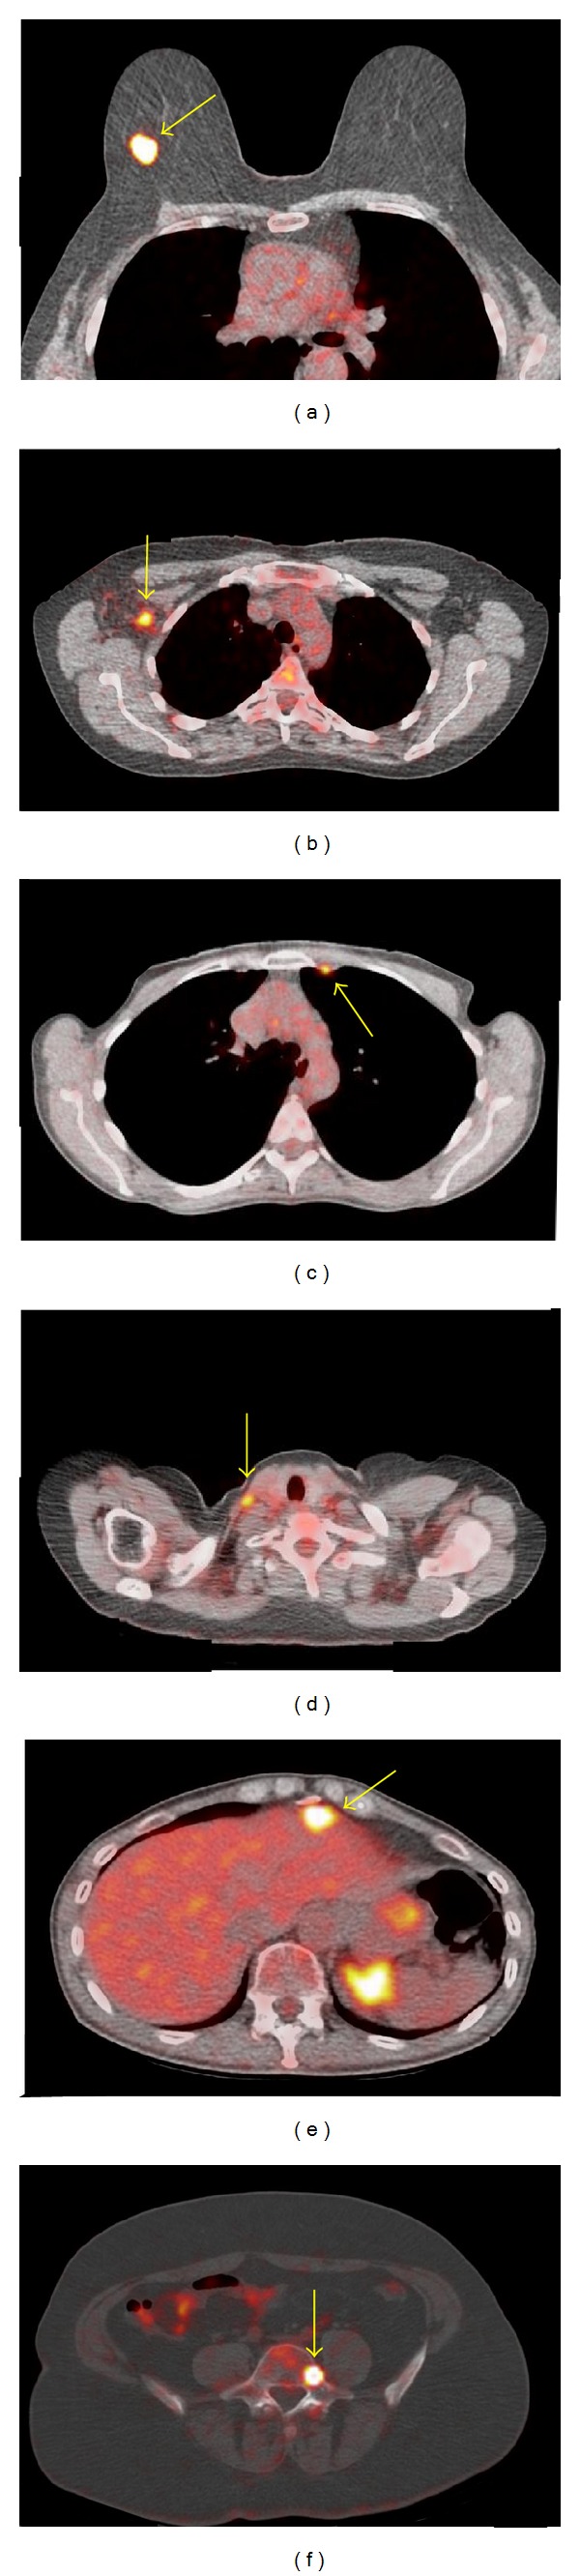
Fused whole-body PET/CT images depicting FDG uptake in the primary tumor (a), an axillary lymph node (b), a lymph node in the internal mammy chain (c), a supraclavicular lymph node (d), the liver (e), and the fifth lumbar vertebra (f).
